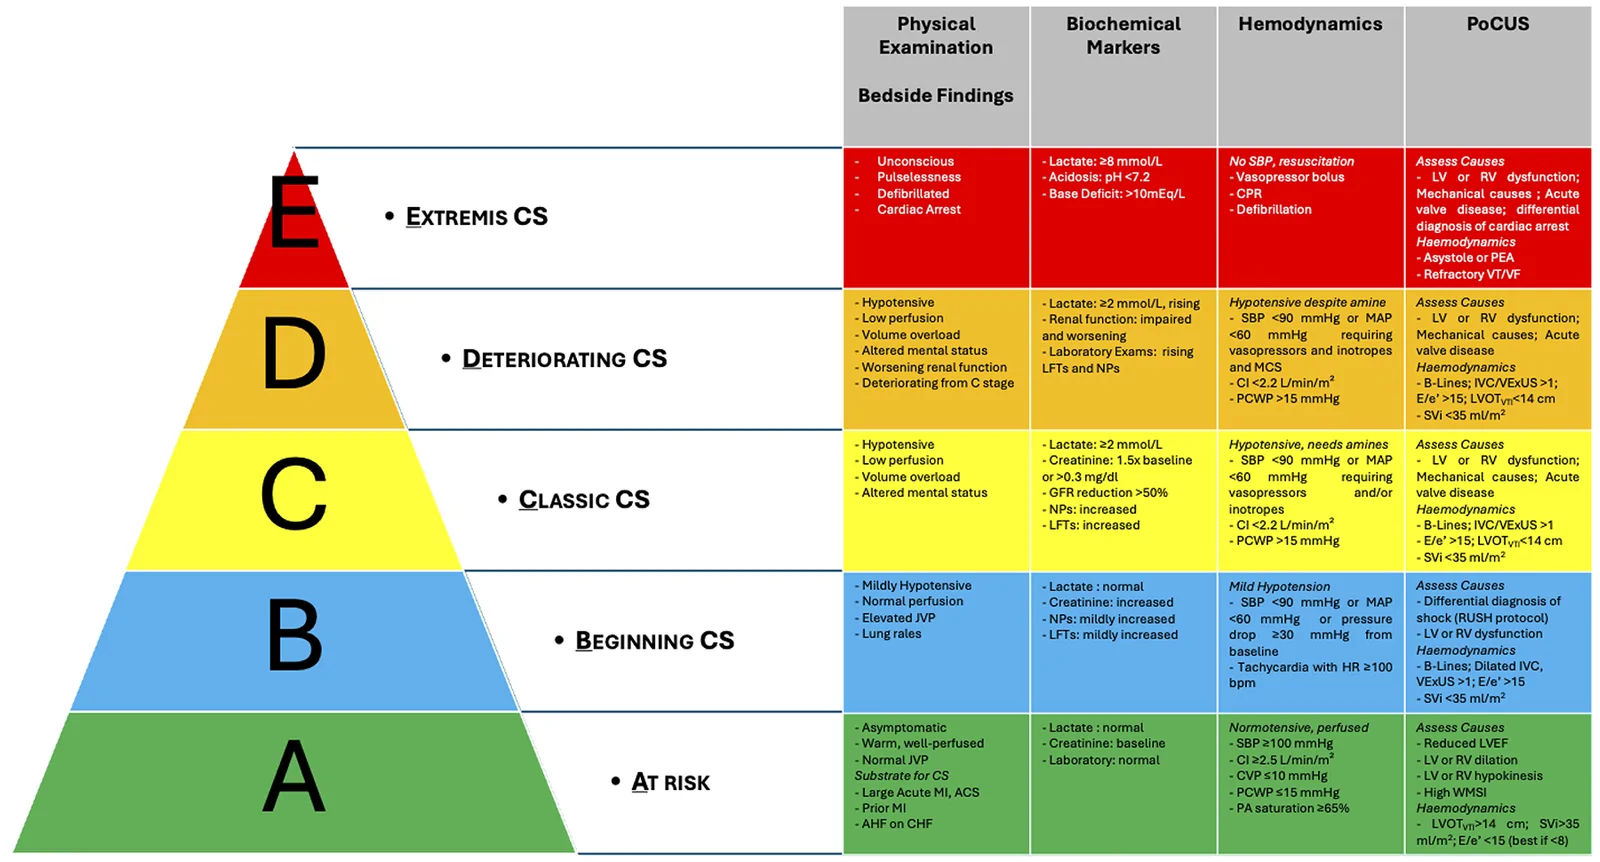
Integration of PoCUS in the SCAI classification. Legend: ACS, acute coronary syndrome; AHF, acute heart failure; CHF, chronic heart failure; CI, cardiac index; CS, cardiogenic shock; CVP, central venous pressure; HR, heart rate; IVC, inferior vena cava; JVP, jugular venous pressure; GFR, glomerular filtration rate; LFTs, liver function tests; LV, left ventricle; LVEF, left ventricular ejection fraction; LVOT_VTI_, velocity-time integral at the left ventricle outflow tract; MAP, mean arterial pressure; MI, myocardial infarction; NPs, natriuretic peptides; PEA, pulseless electrical activity; PCWP, pulmonary capillary wedge pressure; RV, right ventricle; SBP, systolic blood pressure; SVi, stroke volume indexed by BSA; VExUS, venous excess ultrasound; VT/VF, ventricular tachycardia/ventricular fibrillation; WMSI, wall motion score index.
